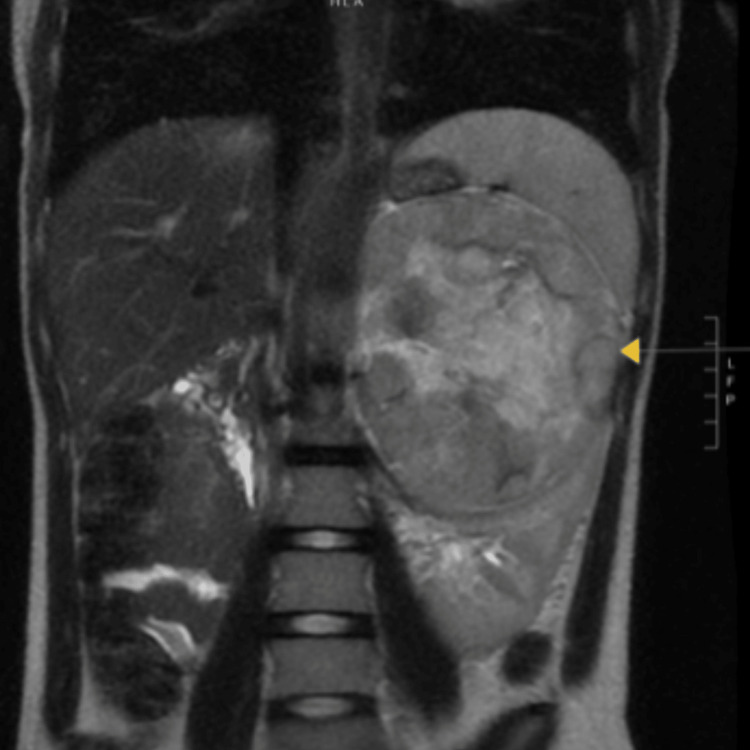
The full body MRI of the patient Yellow arrow points to a 13.9 cm necrotic left adrenal mass
